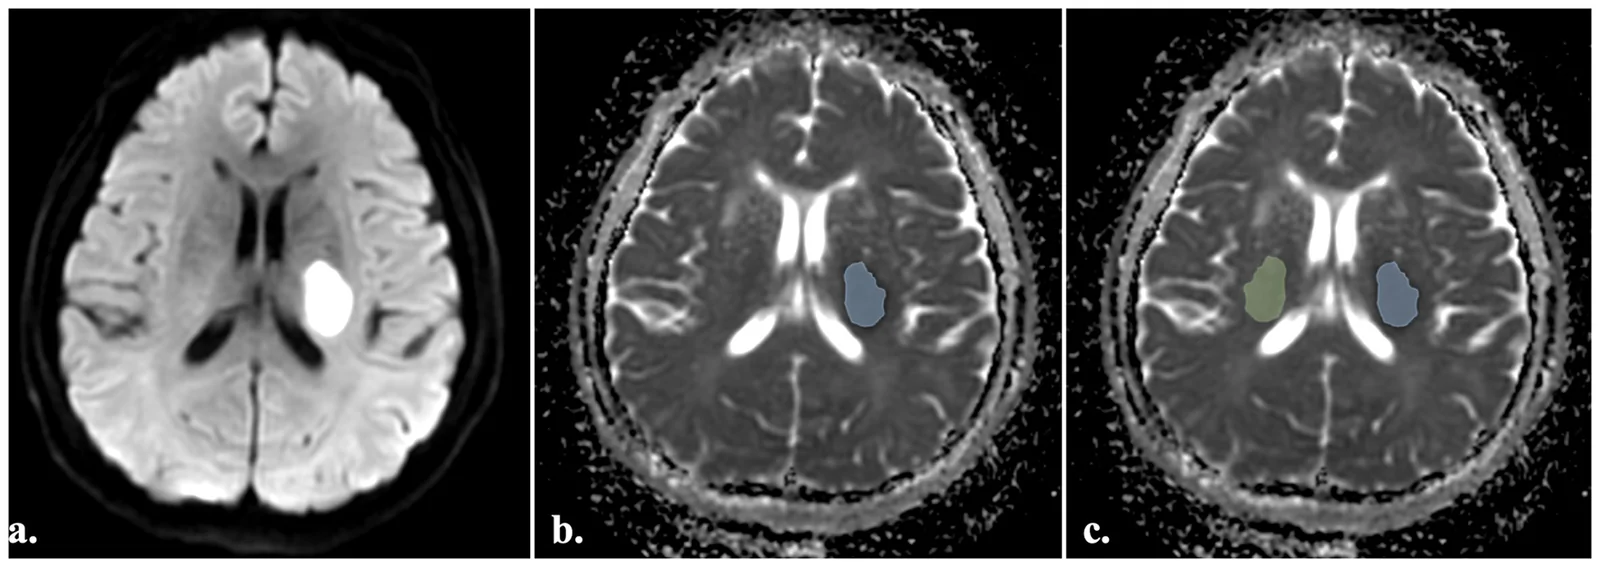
Representative measurement of ADC and rADC values. (a) DWI showing the largest infarct layer. (b) A ROI was manually placed within the ischemic lesion on the corresponding ADC map to measure the ADC value. (c) A mirror ROI was placed in the corresponding contralateral, non-ischemic hemisphere. The rADC was calculated as the ratio of the mean ADC value of the ischemic ROI to that of the contralateral mirror ROI.
